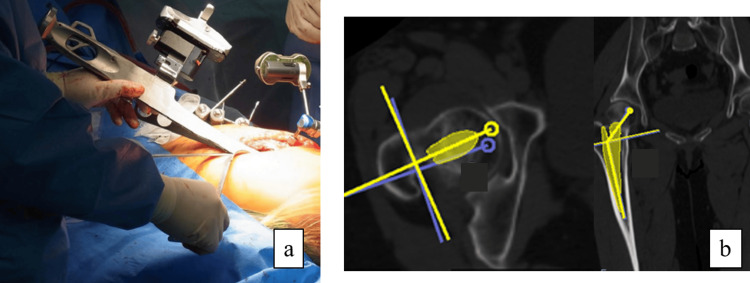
Stem anteversion angle measured using CT-based navigation Intraoperative setup of the CT-based navigation system (a). Postoperative stem anteversion measured using the CT-based navigation workstation (b). CT, computed tomography
